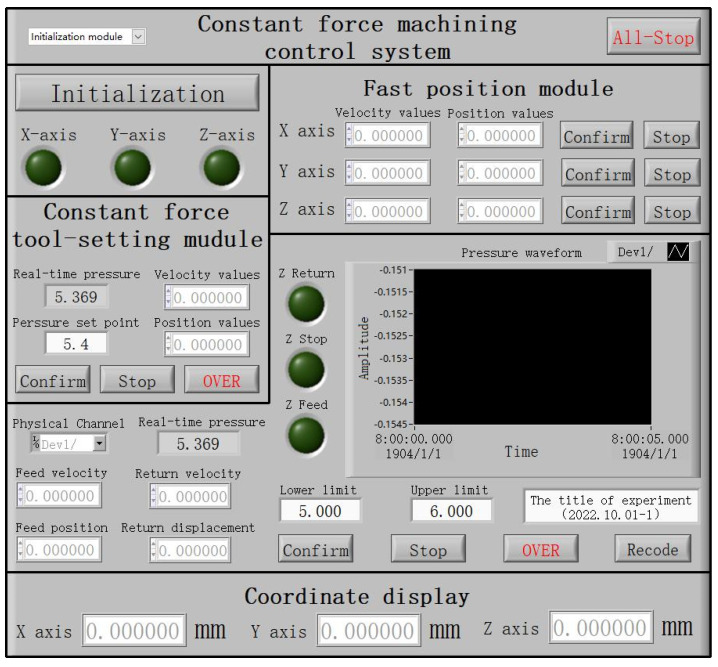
The interface of the template electrophoretically assisted micro-ultrasonic machining control system.
